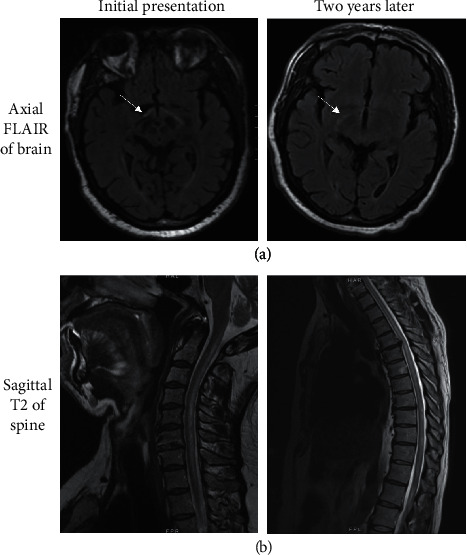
(a) MRI of the orbits showed T2 hyperintensities on FLAIR sequences to the bilateral optic nerves and optic tracts at each presentation (arrows). (b) Spinal MRIs showed longitudinally extensive nonenhancing T2 hyperintensities in distinctly different locations at each presentation.
